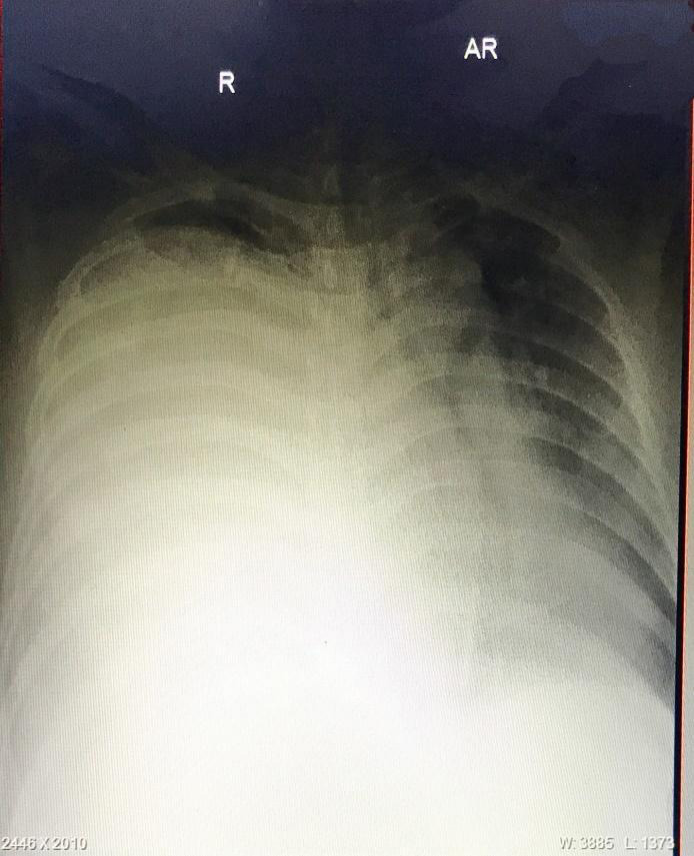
X-ray showing opacity of the right hemithorax.
